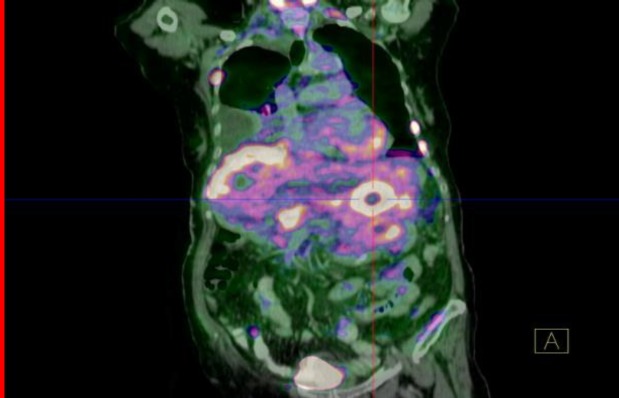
Coronal reconstructed 18F-FDG PET-CT image of the abdomen showing multifocal areas with intense FDG uptake indicating extensive liver and subdiaphragmatic involvement.The lesion in the liver demonstrate peculiar ‘doughnut ‘ appearance indicating centrally located necrotised parenchyma
